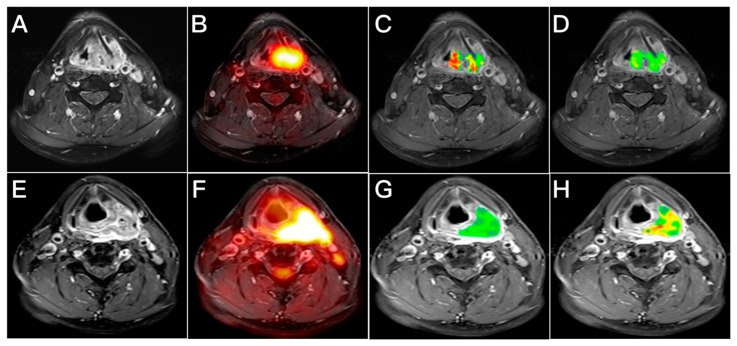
Integrating TN classification with MRI perfusion biomarkers aided in predicting recurrence-free survival. (A–D) Representative axial images of a primary hypopharyngeal cancer patient with T4aN0 disease. (A) Contrast-enhanced MR image; (B) ^18^F-FDG PET/MRI image; (C) Contrast-enhanced MR image with an overlaid *K^trans^* map of the primary tumor; and (D) Contrast-enhanced MR image with an overlaid iAUC map of the primary tumor. This patient had a high *K^trans^* value of 416 × 10^−3^ min^−1^ and a low iAUC value of 424. This patient had local tumor recurrence 12 months after chemoradiotherapy. (E–H) Representative axial images of a primary hypopharyngeal cancer patient with T4aN2b disease. (E) Contrast-enhanced MR image; (F) ^18^F-FDG PET/MRI image; (G) Contrast-enhanced MR image with an overlaid *K^trans^* map of the primary tumor; and (H) Contrast-enhanced MR image with an overlaid iAUC map of the primary tumor. He had a low *K^trans^* value of 117 × 10^−3^ min^−1^ and a high iAUC value of 1044. This patient survived without disease recurrence 5 years after chemoradiotherapy.
